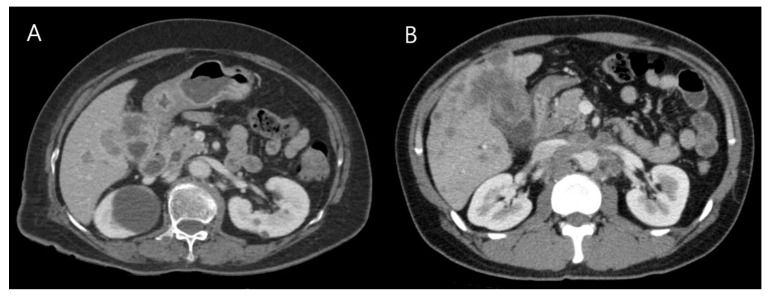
Computed tomography images of gallbladder neuroendocrine carcinoma and adenocarcinoma. (A) Gallbladder neuroendocrine carcinoma with direct invasion and metastases to the liver and regional lymph nodes. (B) Gallbladder adenocarcinoma with direct invasion and metastases to the liver and regional lymph nodes.
